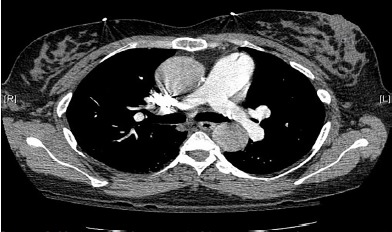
Unremarkable computed tomography pulmonary angiogram.
